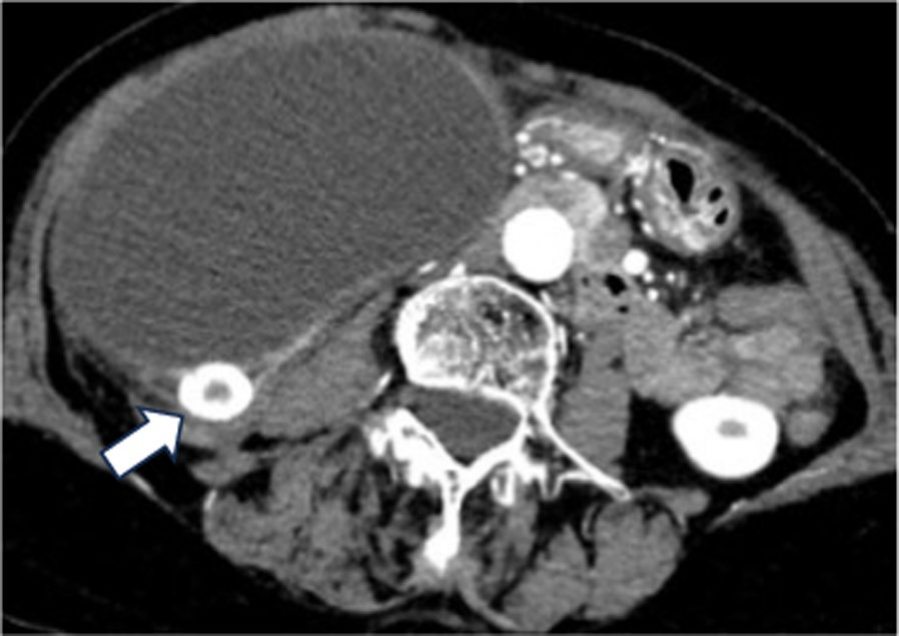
A contrast-enhanced CT scan of the abdomen revealed a grossly distended gallbladder (14.5 × 14.5 × 8.7 cm) containing a round stone (white arrow)
